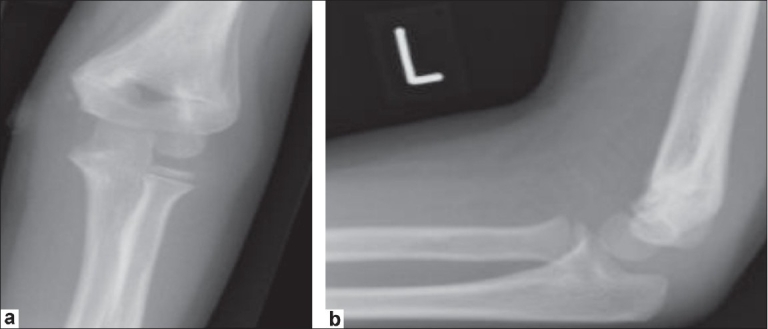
(a) Anteroposterior radiograph demonstrating the medial epicondyle in an anatomical position with union of the distal humerus. (b) Lateral radiograph demonstrating the medial epicondyle in an anatomical position with union of the distal humerus
